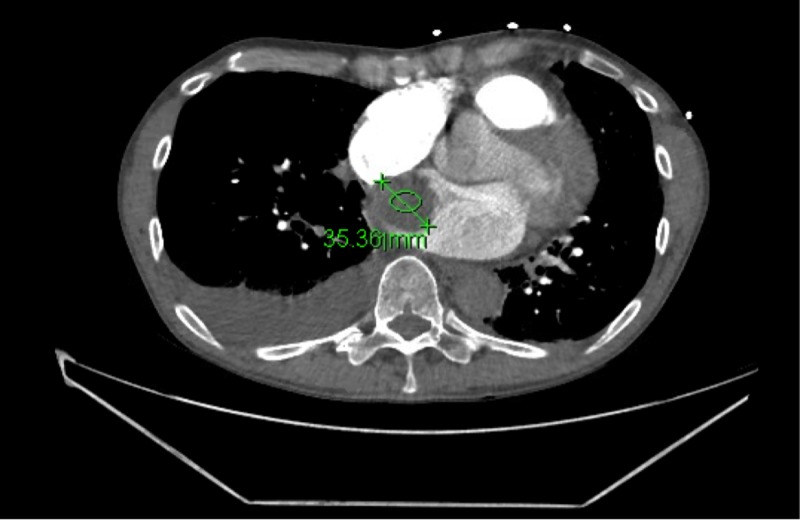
CT scan to rule out pulmonary embolism showing a 35.36 mm right atrial cardiac tumor.
